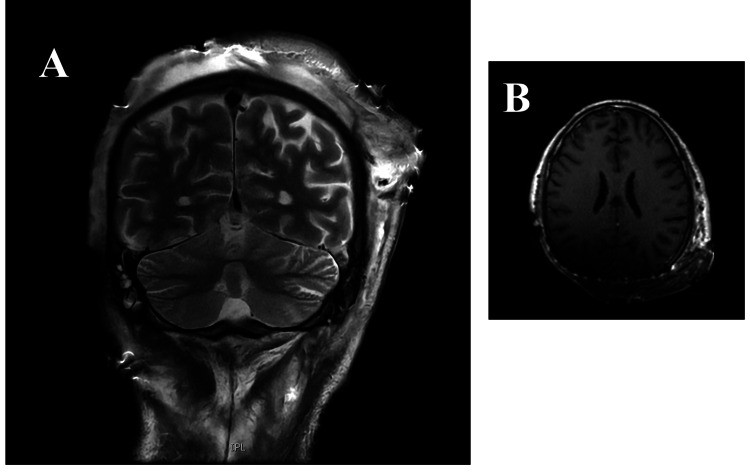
Postoperative MRI imaging of the brain: axial T1-weighted (A) and coronal T2-weighted (B) sequences after gadolinium administration showing residual enhancing lesion next to the titanium mesh in the left parieto-occipital region MRI: Magnetic resonance imaging.
